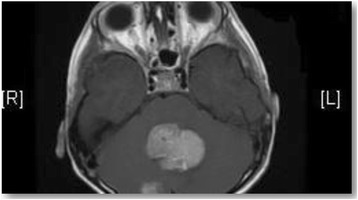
Brain MRI revealed a posterior fossa tumor with obstructive hydrocephalus
